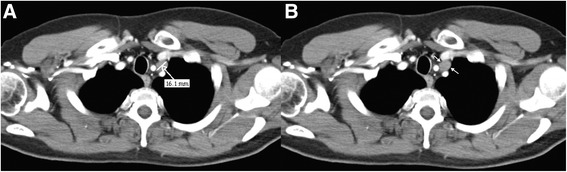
a, b Full-body computed tomography scan showing a 16-mm lymph node under the left collarbone (*arrows*)
